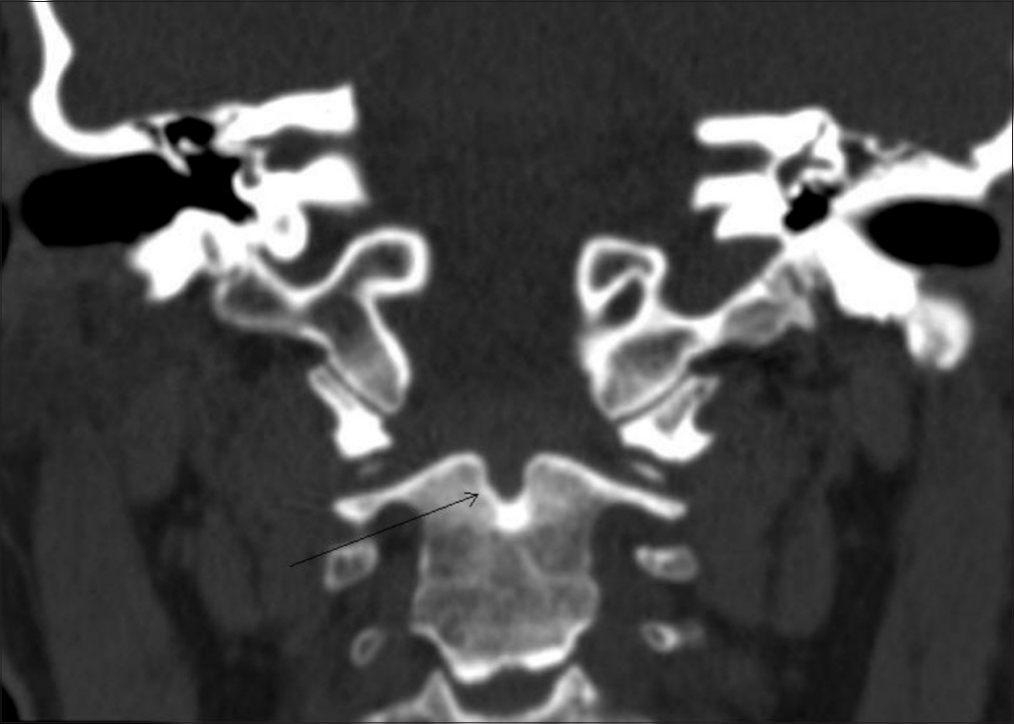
Coronal CT scan reconstruction shows a well-corticated depression (arrow) on the superior aspect of the body of C2, with non-visualization of the dens
